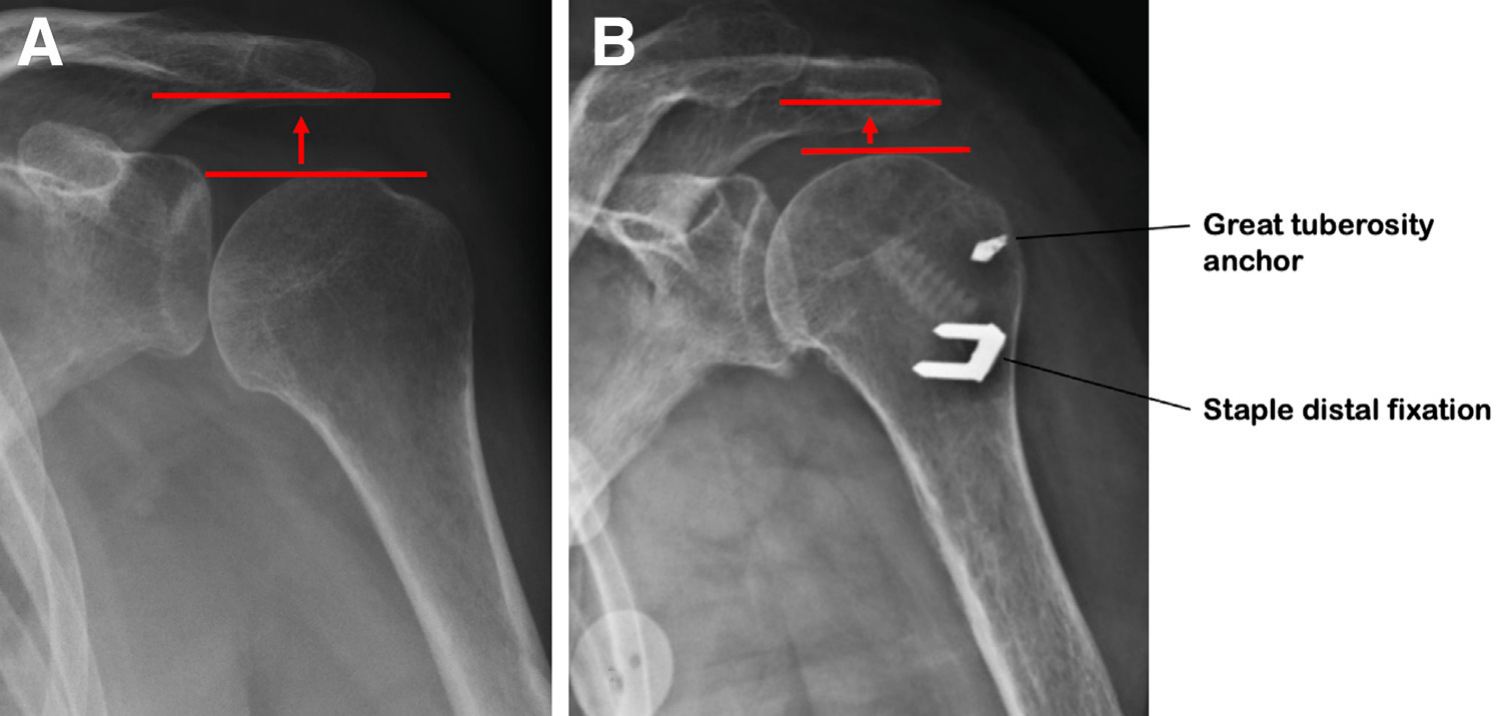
Anteroposterior radiograph of a left shoulder showing inferior subluxation with abnormal gap between the inferior aspect of the acromion and the superior aspect of the humerus (a). Postoperative anteroposterior left shoulder radiograph showing narrowing subacromial space with staple and anchors int the superior part of the humerus (b).
